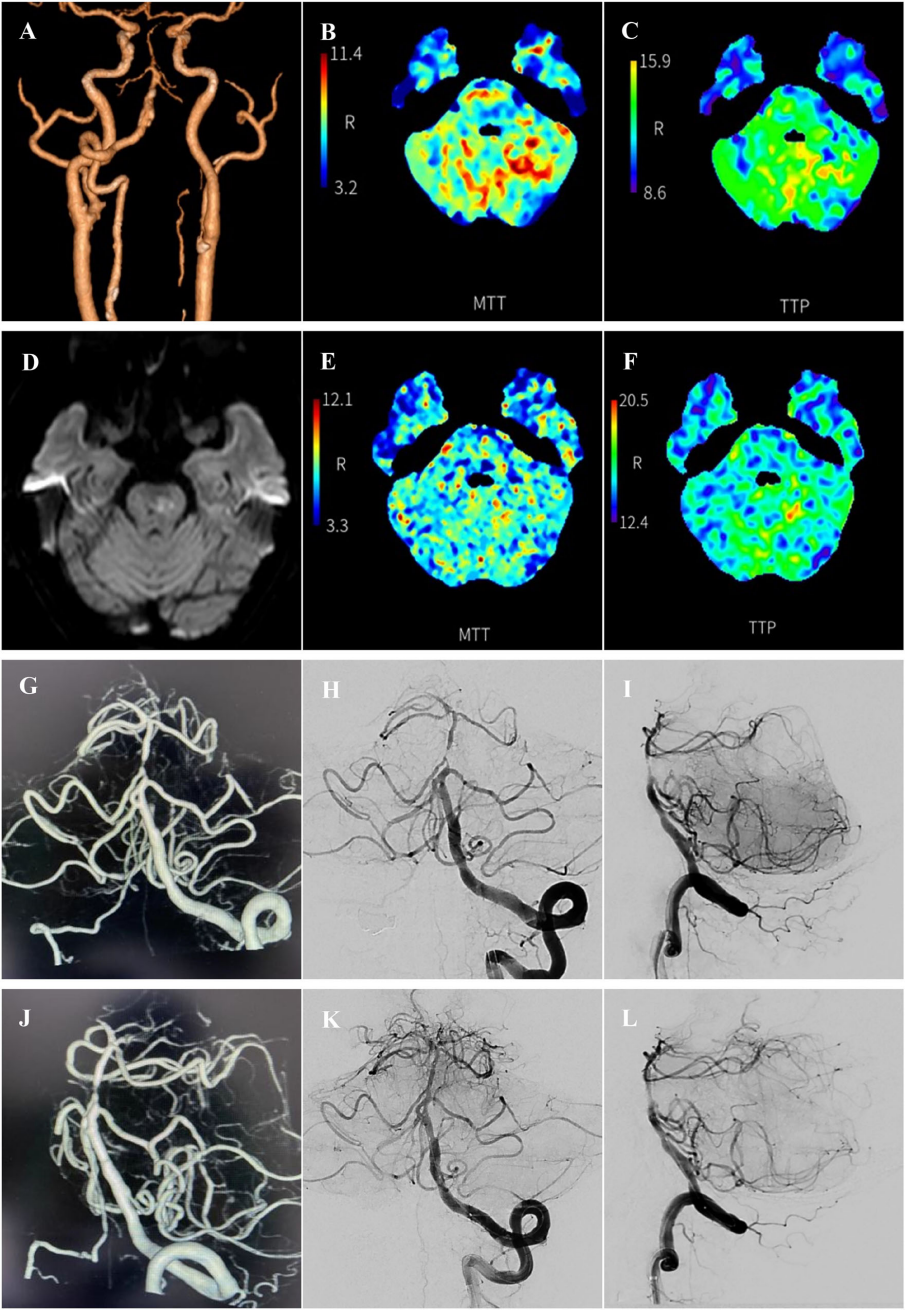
A case of sICAS in BA. (A–C): Preoperative CTA and CTP revealed severe stenosis of BA and the brain tissue it innervates was hypoperfused. (D): New infarction of brainstem was observed in the MRI. (E and F): Postoperative CTP showed that the corresponding cerebral tissue hypoperfusion was significantly improved. (G–J): Preoperative DSA showed severe stenosis of the lumen of BA, and the distal branches were sparse. (K and L): After angioplasty, a Neuroform Atlas stent was successfully implanted in the BA, and follow‐up angiography 10 min later showed good blood flow. BA, basilar artery; CTA, computed tomography angiography; CTP, computed tomography perfusion; DSA, digital subtraction angiography; MRI, magnetic resonance imaging; sICAS, symptomatic intracranial atherosclerotic stenosis.
